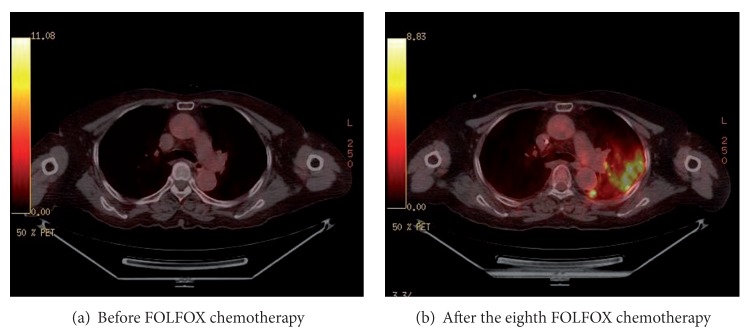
PET-CT after the eighth cycle of chemotherapy.
